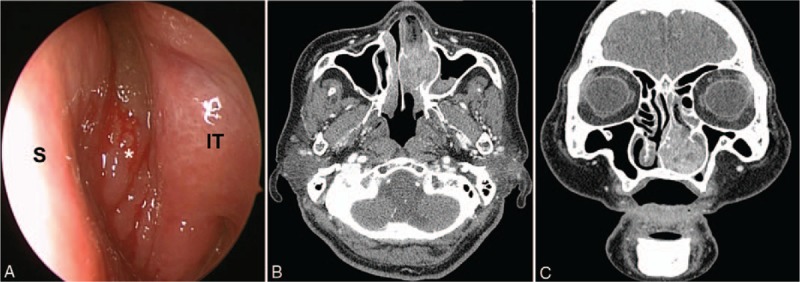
Preoperative imaging. (A) Nasoendoscopy reveals a polypoid mass (asterisk) causing bleeding on touch in the left nasal cavity. (B) Axial and (C) coronal contrast-enhanced computed tomography of the paranasal sinuses shows a 3.7-cm enhancing and expanding lesion in the left nasal cavity with accompanied remodeling of the nasal septum and inferior turbinate. IT = inferior turbinate, S = septum.
